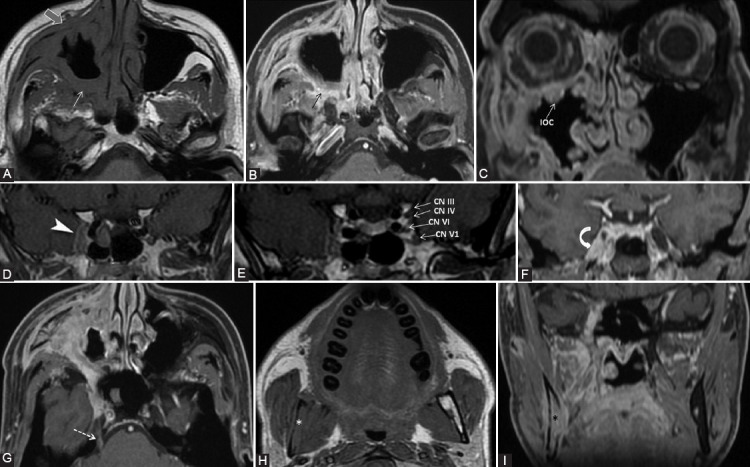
A 39-year-old male diagnosed with adenoid cystic carcinoma of maxillary sinus, with multiple extensions. Clinically: Right abducens nerve palsy, diplopia, right infraorbital anesthesia. Axial T1-weighted (A, H), axial (B, G), and coronal (C, F, I) contrast-enhanced (CE) 3D T1 FSPGR; coronal contrast enhanced in Phase T1 FSE IDEAL (D, E) images. The probable first point of PNS is PPF with fat obliteration (white arrow) and CE (black arrow), where the V2 nerve is involved. In an anterograde direction, the tumor reaches the right infraorbital nerve, demonstrated by infraorbital canal enlargement with CE (IOC) and preantral fat obliteration (gray arrow). Following the main V2 trunk, the tumor reaches the cavernous sinus which appears larger and shows CE (arrowhead). Note that in CN III, IV, V1, and V2 are situated in the lateral wall of the cavernous sinus, the cavernous segment of CN VI is located medially to the CN V1, implying the tumoral involvement of the mentioned nerves. There is Meckel’s cave CE (curved arrow) and the tumor traveled back up to the main trigeminal trunk (cisternal segment, dashed arrow). Notice the right mandibular fat replacement (white *), associated with mandibular CE (black *), and cortical disruption, meaning an anterograde PNS from the Meckel’s cave to the inferior alveolar nerve (branch of V3).
